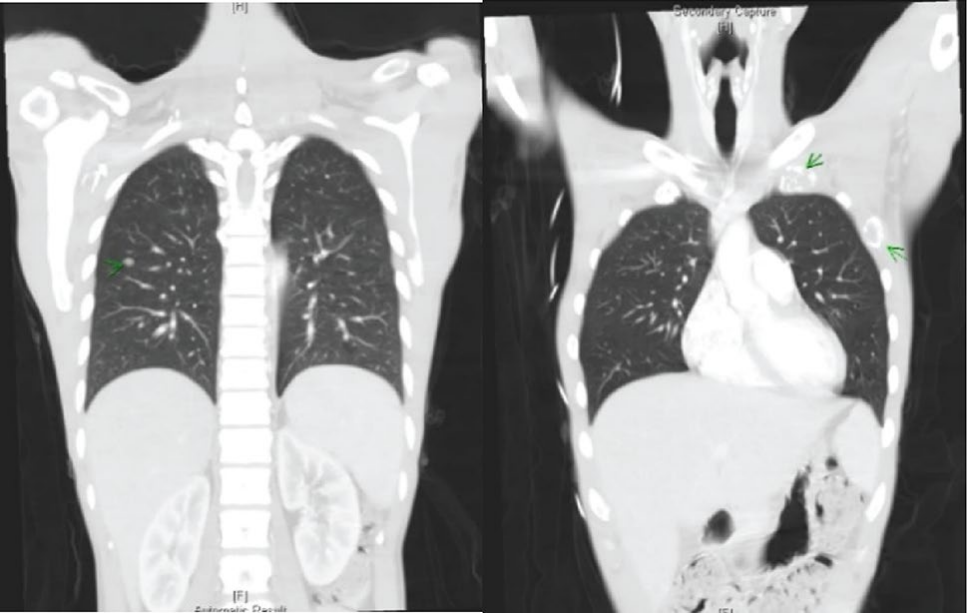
Chest computed tomography (CT) scan revealing a 0.85 cm ovoid solid nodule in the right lower lung, along with multifocal osteolytic bony lesions observed in the sternum, left 1st, 3rd ribs, and right humeral head
